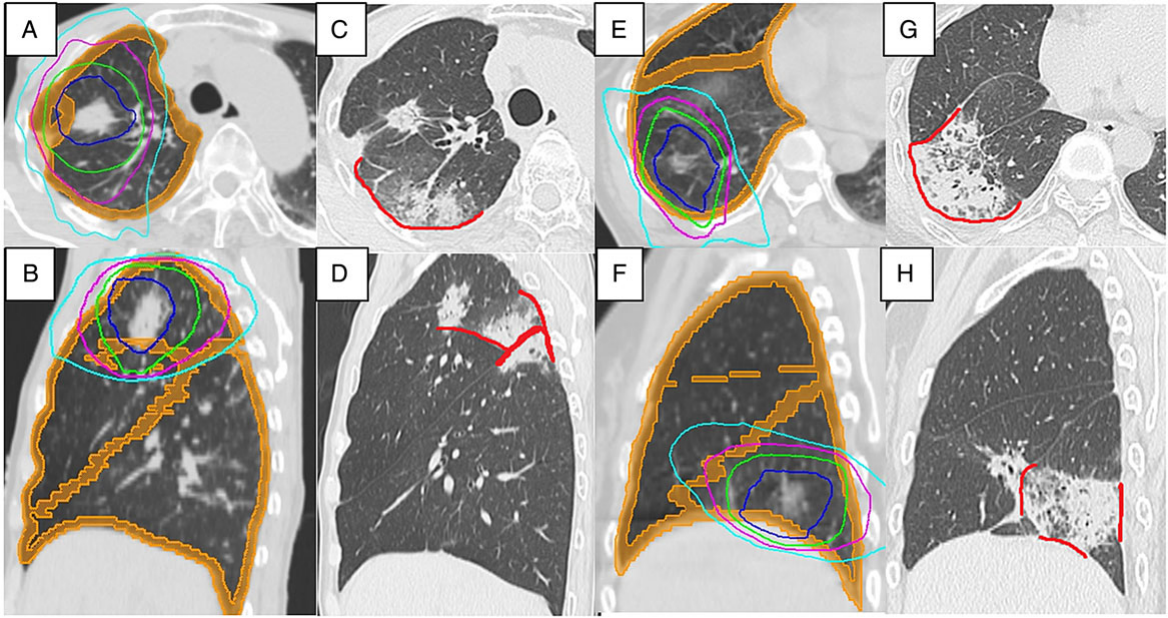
Dose distribution of stereotactic body radiotherapy (SBRT) for a tumor in superior (A, B) and in inferior lobe (E, F). Prescribed doses were both 48 Gy in four fractions. Isodose lines of 45 Gy (blue), 40 Gy (green), 30 Gy (purple) and 20 Gy (light blue) are shown. Orange regions represent subpleural region of the lung or pleura. Chest computed tomography (CT) scans at 3 months after SBRT are shown (C, D, G, H). The red lines represent the lesions where the radiation pneumonitis (RP) attached to the pleura. Both patients developed symptomatic RP. Compared with the patient with a tumor in the inferior lobe (G, H), the RP lesion attached more extensively to the pleura in the patient with a tumor in the upper lobe (C, D). The patient with a tumor in the upper lobe developed OP 6 months after SBRT. The RP of another patient with a tumor in the lower lobe was resolved without any relapse.
